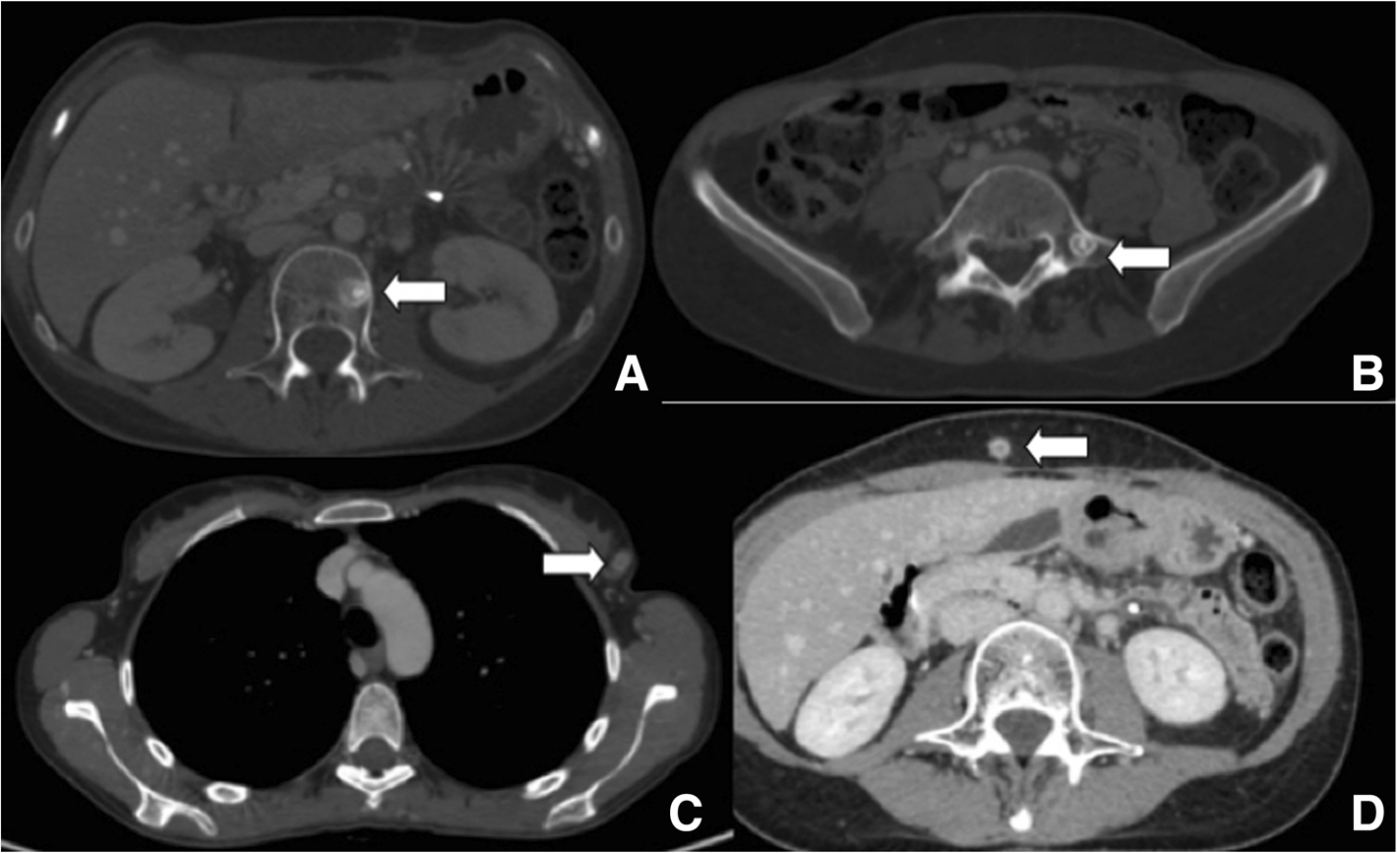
CT images at the time of systemic disease progression showing bone metastases in L3 (a) L5 (b) vertebra, left breast (c), and subcutaneous tissues of the abdominal wall (d)
